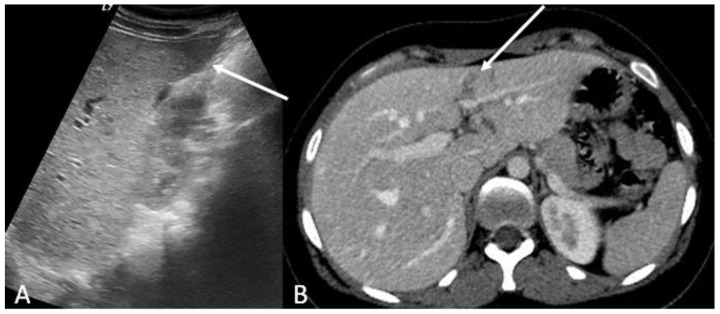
US (A) and enhanced-CT (B, venous phase) of a 32 year old male who sustained major trauma. US scans of the liver shows a subtle hypoechoic area (A, arrow). Enhanced-CT allows exhaustive evaluation of the suspected liver injury, depicting the whole extension of the liver laceration (B, arrow) and excluding the presence of vascular injuries, thus allowing safe conservative management of the patient.
